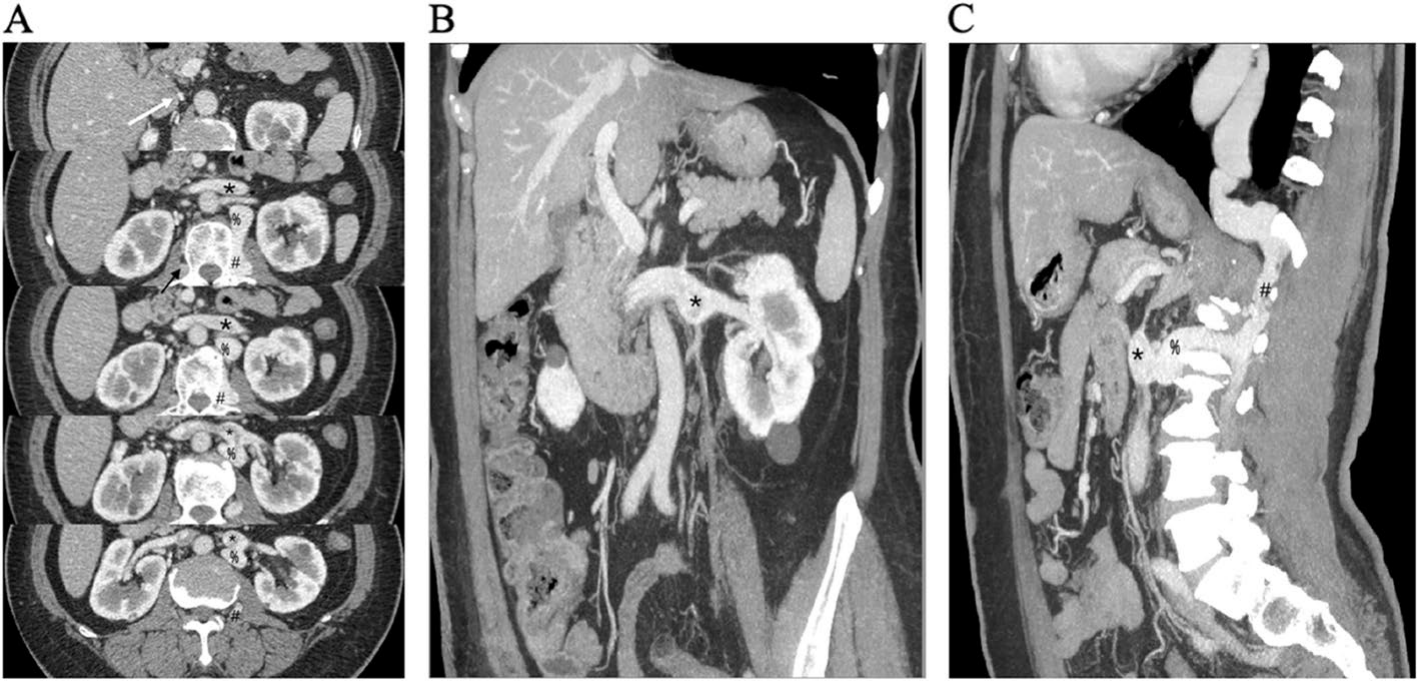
Contrast-enhanced abdominopelvic CT images of the patient in rest state. A. Cross-sectional images shows localized enlargement of LRV (*) and tortuous dilatation of the right ascending lumbar vein (black arrow), left ascending lumbar vein (#), and bilateral lumbar veins. There is no sign of nutcracker phenomenon. The white arrow indicates the blind end of infrarenal IVC. B. Oblique coronal MIP image at delayed phase shows local dilatation of left renal vein (LRV) (*) near the hilum. C. Sagittal MIP image at delayed phase shows that LRV (*) is connected with left ascending lumbar vein and hemiazygos vein (#) via a communicating branch (%), and all of the vessels are dilated. MIP, maximum intensity projection
